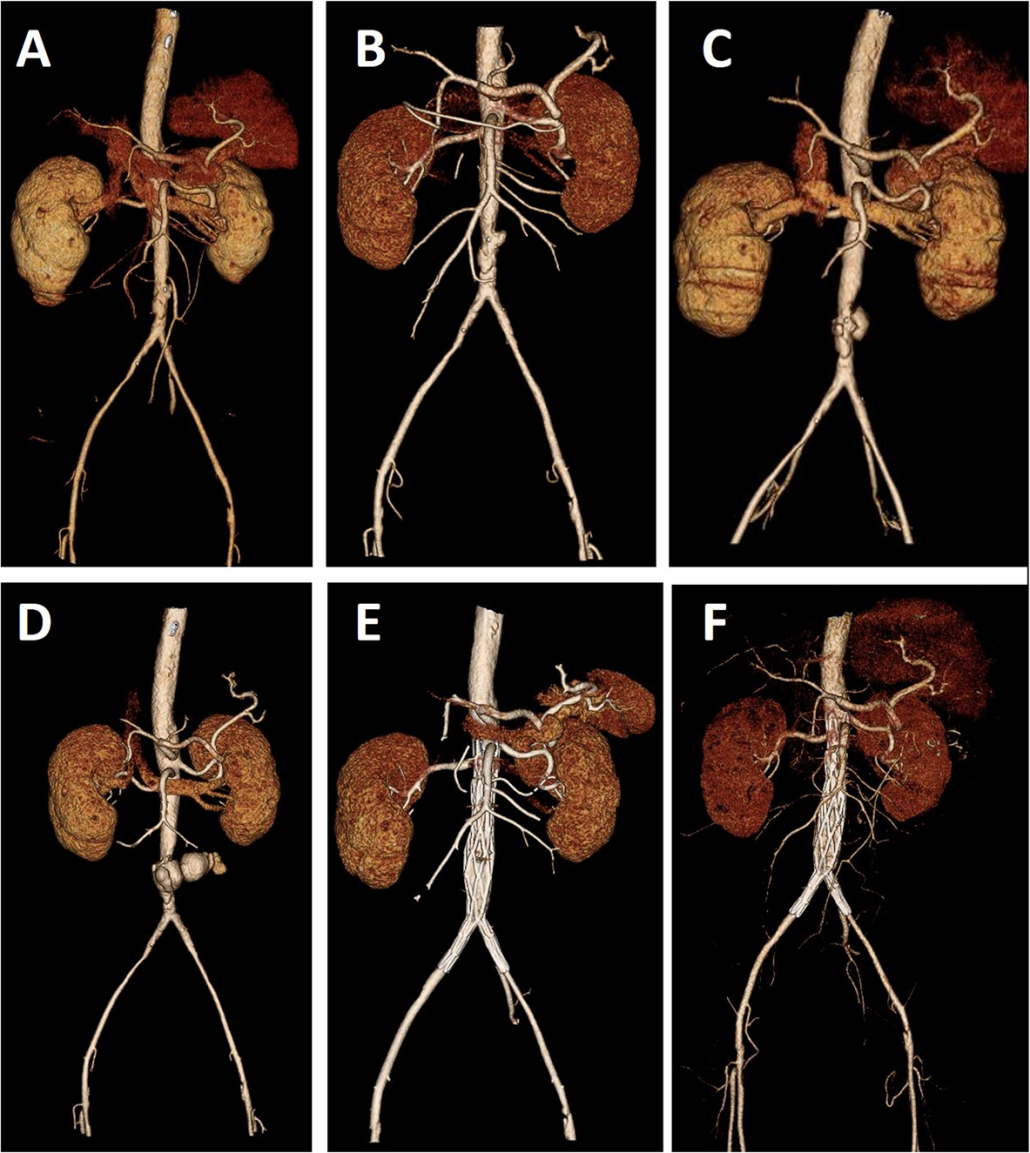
CTA reconstruction of the infrarenal abdominal aorta on day 1 (a), 5 (b), 21 (c), 40 (d), 14 days after EVAR (e) and 1 year after operation (f), showing the dynamic changes of infectious aortic aneurysm. CTA = computed tomography angiography
